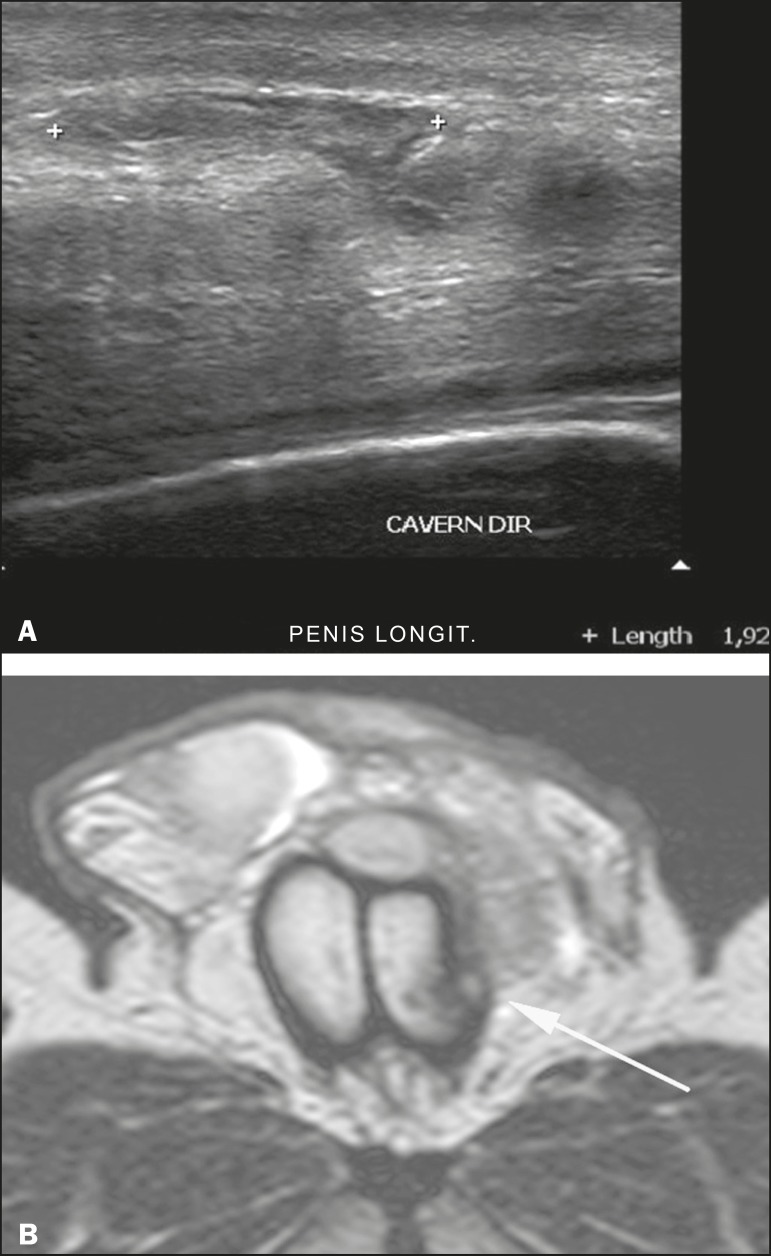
A: Ultrasound of the penis, right lateral view. Longitudinal
